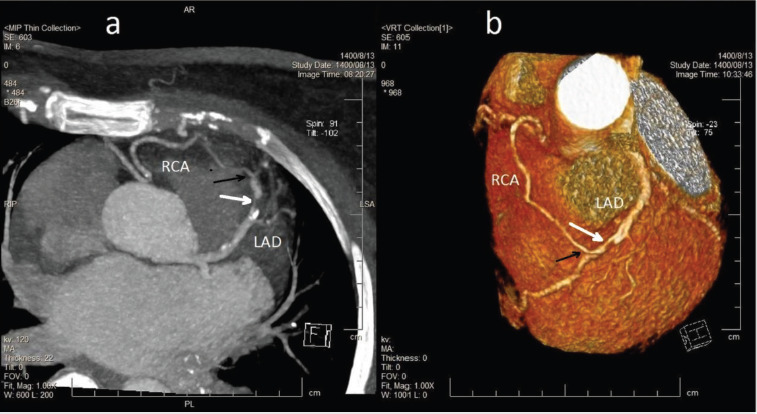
(a) Maximum intensity projection and (b) volume rendering technique reconstruction images from coronary *Multi-Detector Computed Tomography* (MDCT). Coronary MDCT reveals the anomalous origin of the right coronary artery (RCA) from the mid-left anterior descending artery (LAD), distal to a stenotic segment. Stenosis was severe (70–99%) in the LAD. White arrows indicate the site of stenosis in the LAD, whereas black arrows point to the anomalous origin of the RCA from the LAD.
